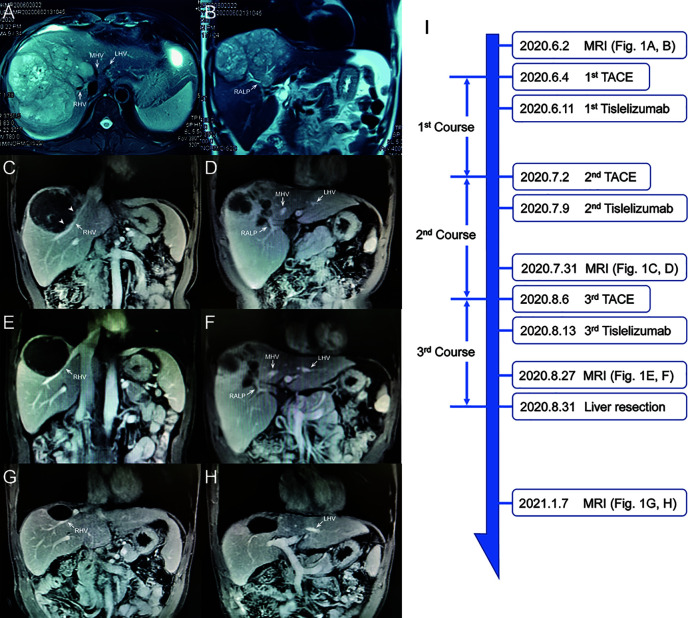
Abdominal MRI scan at different treatment phases. (A, B) Images at initial diagnosis. (C, D) Images after 2^nd^ course. Arrowheads indicate viable tumor lesions near the RHV. (E, F) Images before surgery. (G, H) Images at 4-month after surgery. (I) Timeline of treatment process. RHV, right hepatic vein; MHV, middle hepatic vein; LHV, left hepatic vein; RALP, right anterior lobe pedicle.
